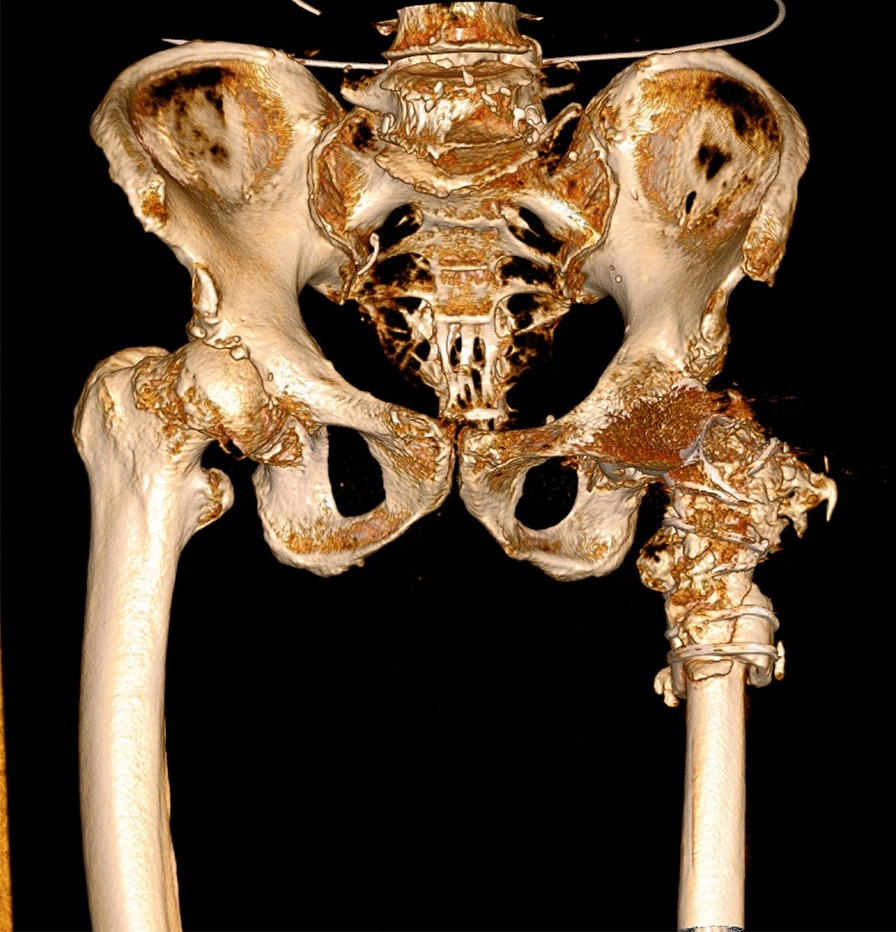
3D computed tomography reconstruction model of the pelvis and hip at 10-month follow-up postoperatively
